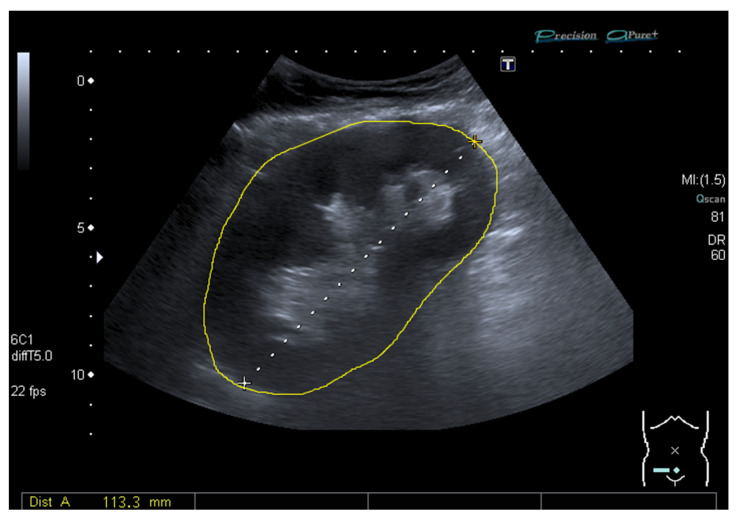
B-mode image from a common ultrasound of a transplanted kidney manually selected for calculation of the grayscale median (50).
